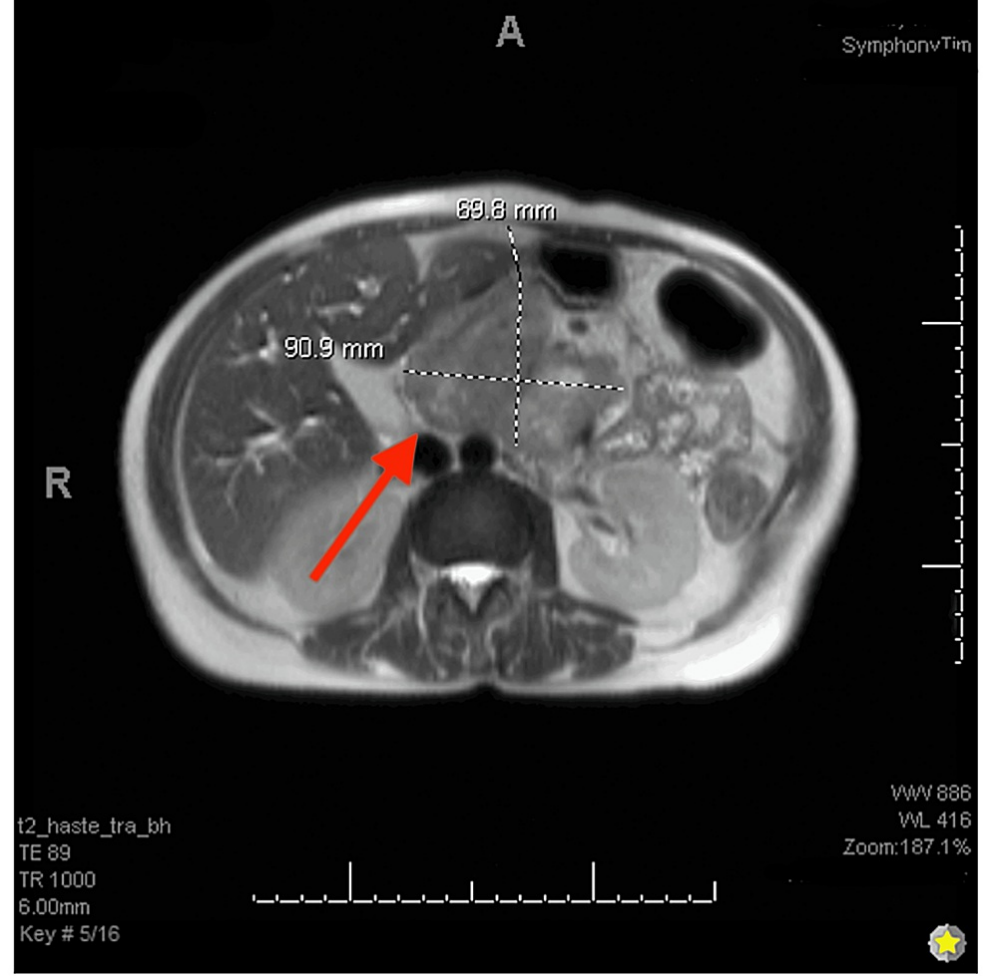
T2-weighted MRI abdomen with and without MRCP (axial view) showing pancreatic mass post-gadolinium contrast administration. MRCP: magnetic resonance cholangiopancreatography
